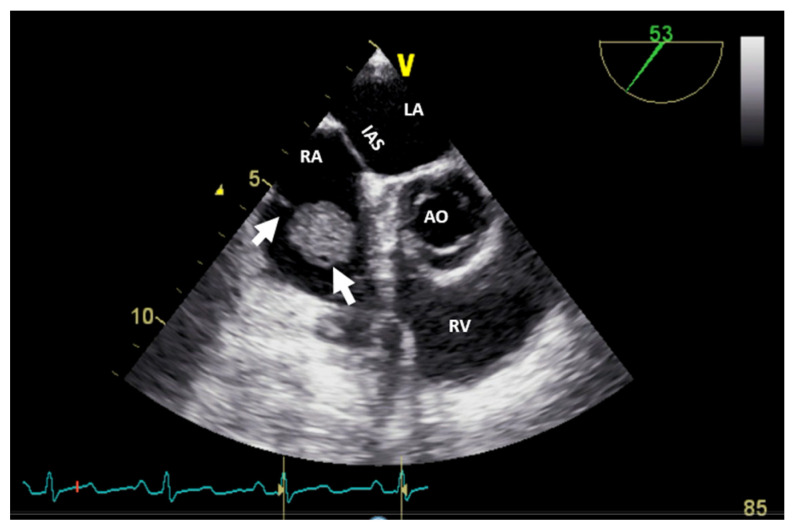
Two-dimensional transesophageal echocardiography (short-axis view at the level of the great vessels) showing a mass (arrow) on the port-a-cath in a patient with neoplasia and chemotherapy. It was an incidental finding. The mass disappeared after initiating anticoagulation treatment. AO, aorta; IVS, interventricular septum; LA, left atrium; LV, left ventricle; RV, right ventricle.
